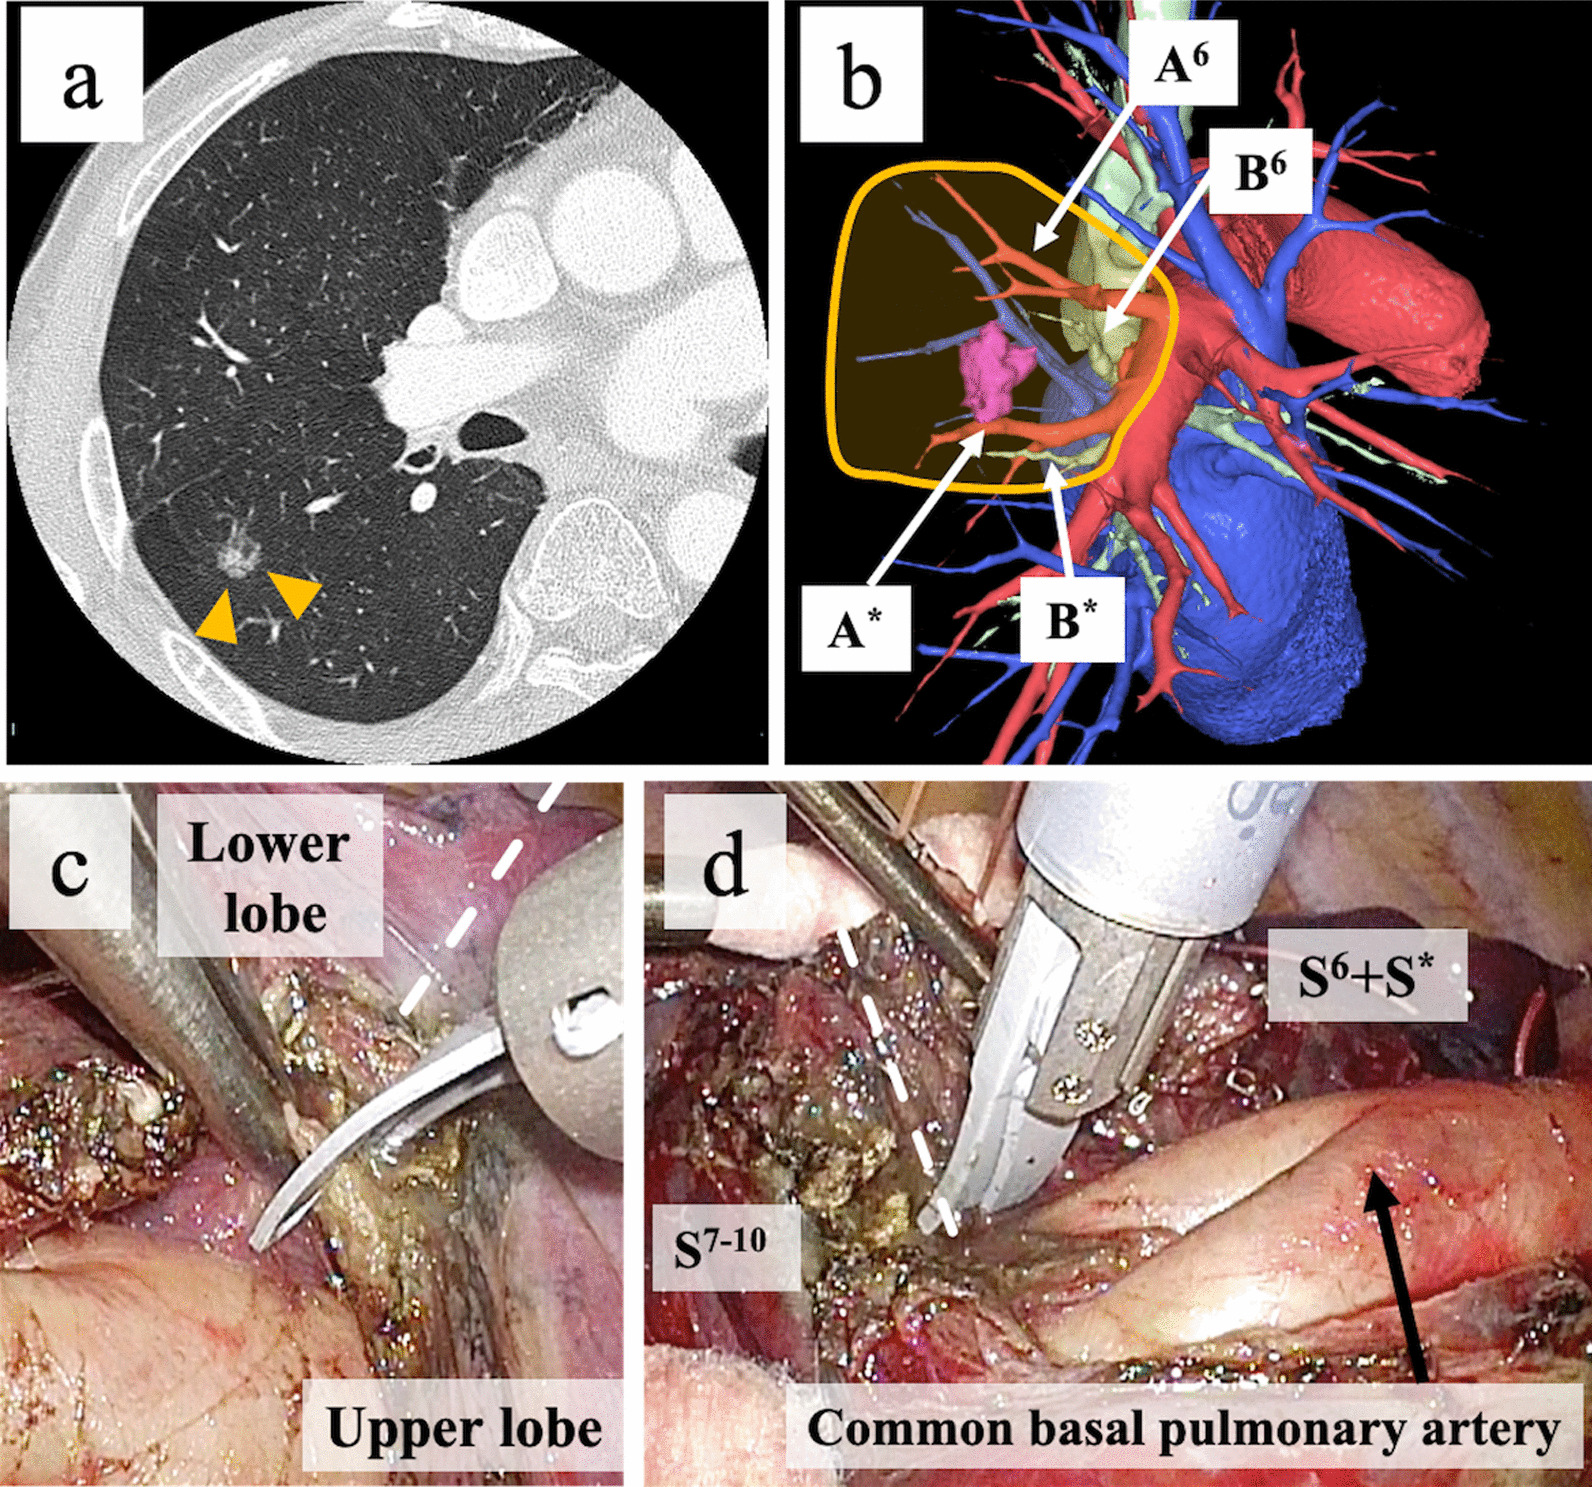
Segmentectomy (the S^6^ + S* segment) for lung cancer with incomplete lobulation. (a) High-resolution CT and (b) three-dimensional CT images showing a pulmonary nodule in the right S^6^ + S* segment. The yellow box indicates the area to be resected. (c) The interlobar lung parenchyma with incomplete lobulation between the right upper and lower lobes (white dotted line) is dissected using the microwave surgical instrument toward the second carina. (d) The S^6^ + S* segment including the tumor emerges sufficiently from the pulmonary hilum and is subsequently sectioned off between the intersegmental plane (white dotted line) by an ASI. S^6^, superior segment; S*, subsuperior segment; CT, computed tomography
